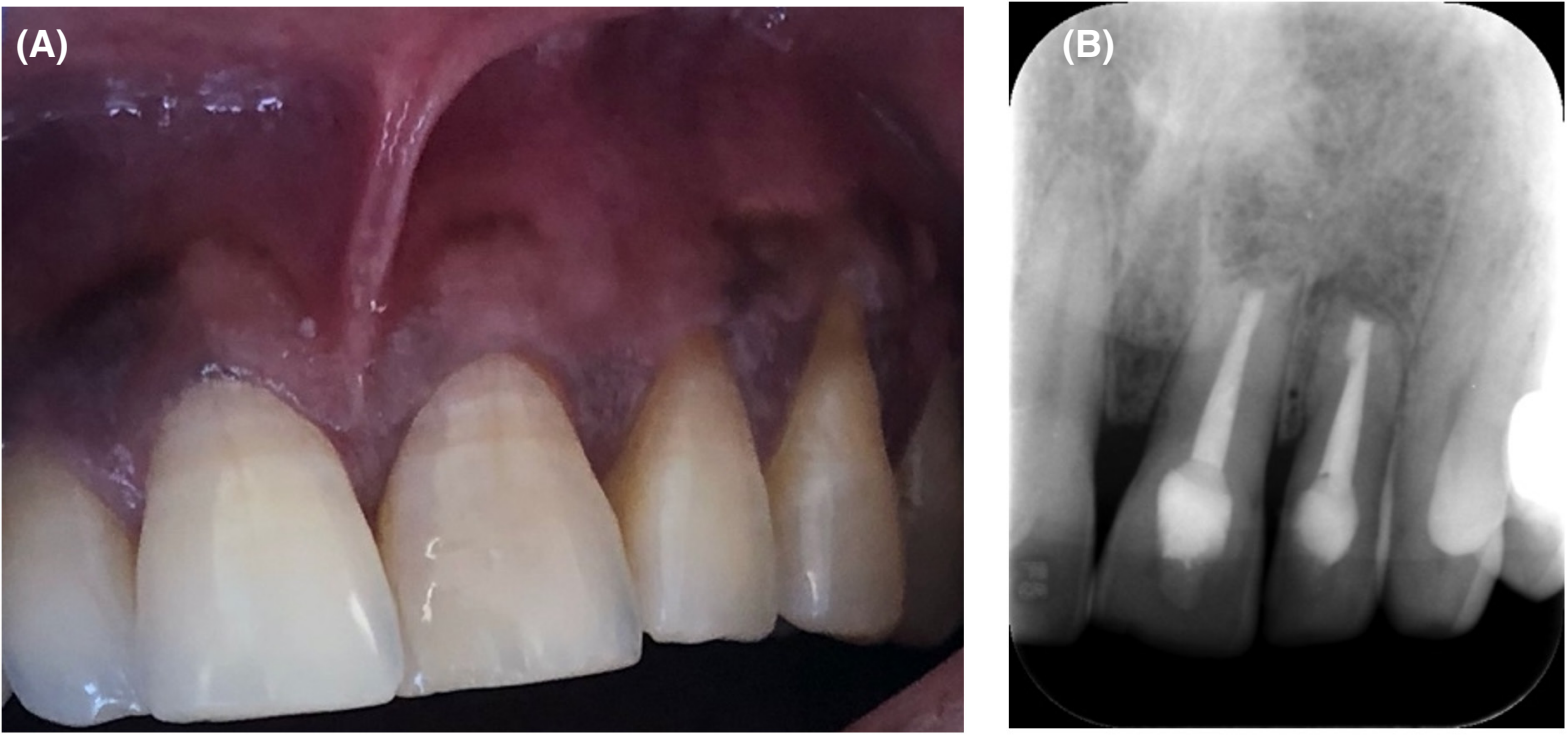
(A) Soft tissue healing, (B) 2 years follow up periapical radiograph.
